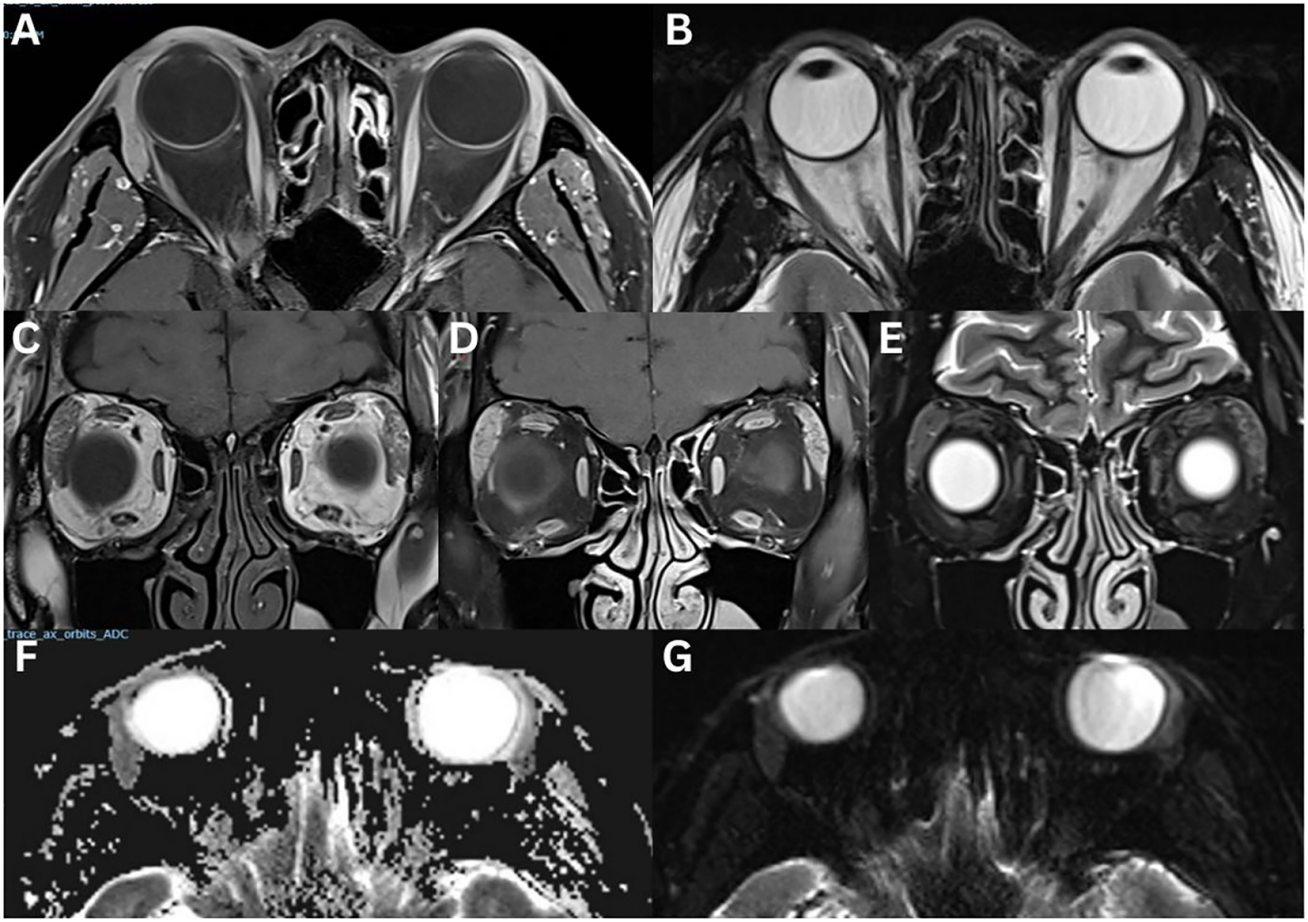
Thyroid eye disease with asymmetrical bilateral lacrimal gland involvement. MRI T1-weighted axial fat-suppressed contrast-enhanced (A), T2-weighted axial (B), T1-weighted coronal (C), coronal fat-suppressed contrast-enhanced (D), and T2-weighted coronal (E) sequences demonstrate bilateral enlargement of the lacrimal glands, with moderate enhancement following contrast administration. The glands appear mildly hyperintense on T2-weighted imaging, relative to the adjacent extraocular muscles. There is a restricted diffusion pattern on axial ADC (F) and axial TRACE (G).
